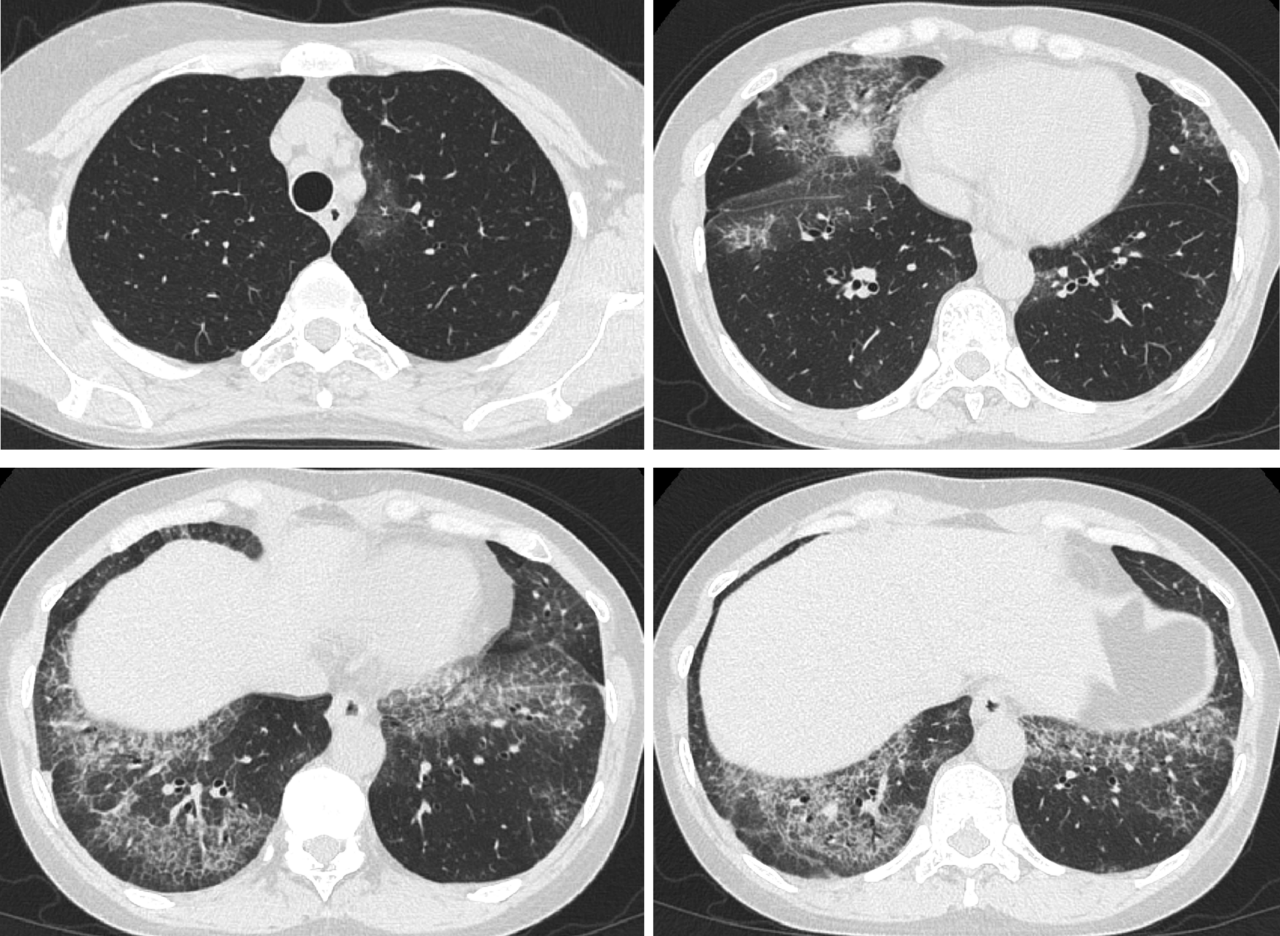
Thin‐section computed tomography of the lung showing bilateral patchy ground‐glass opacifications with interlobular septal thickening and intralobular lines, predominantly in the lower lobes (“crazy paving” appearance).
